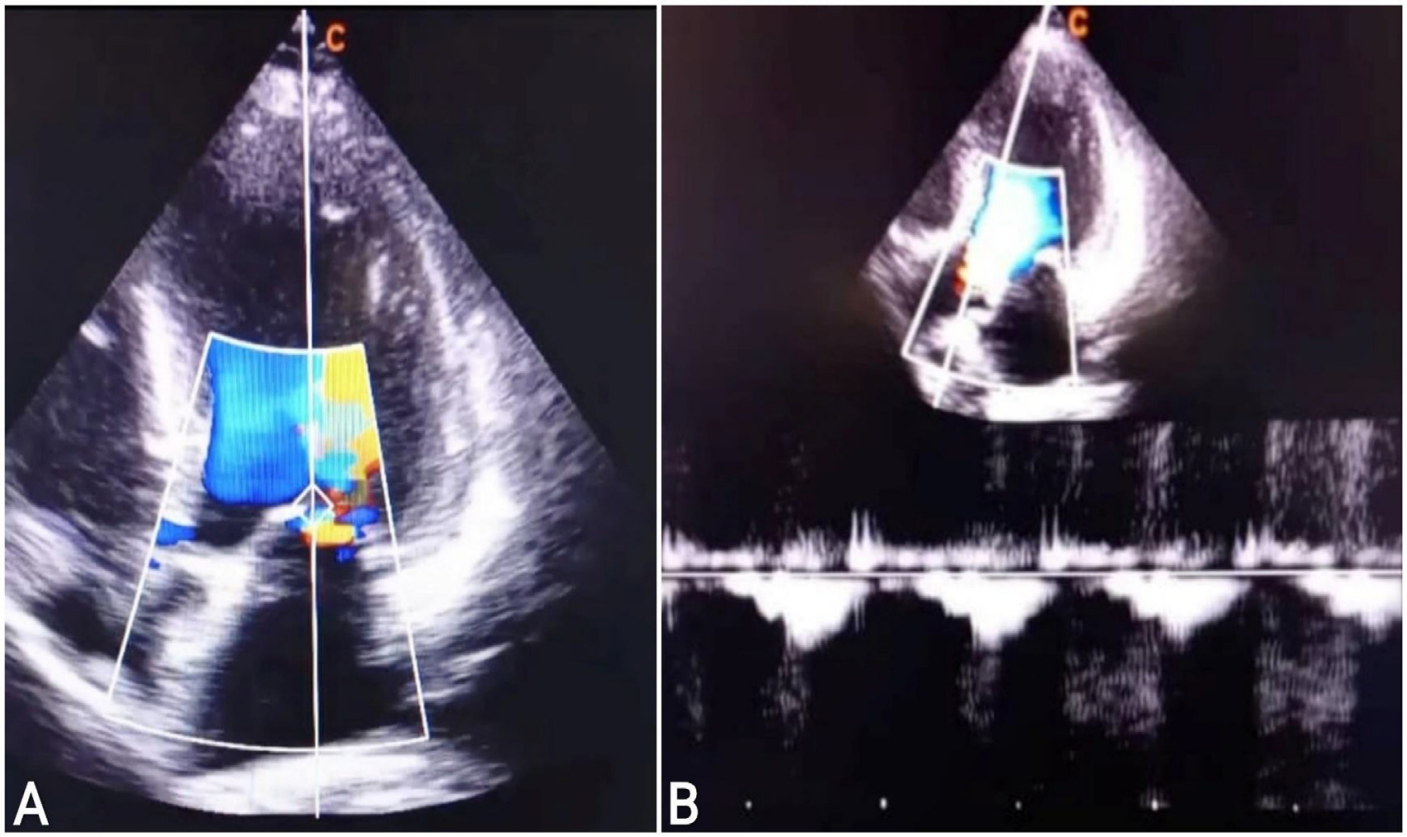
The two-dimensional transthoracic echocardiography. (A): The apical 4-chamber view echocardiogram displayed mild mitral regurgitation. (B): Continuous wave doppler of the normal aortic valve without stinosis or regurgitation.
